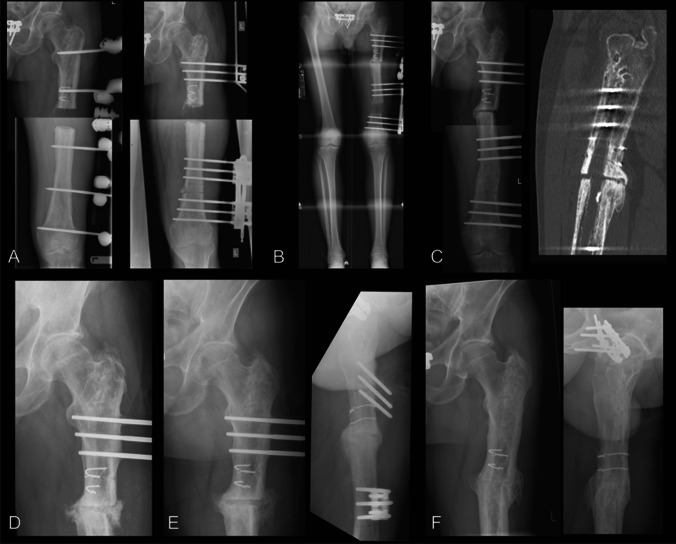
Docking site non-union in patient treated for infected non-union of femur managed by bone grafting. A Initial radiographs following removal of femoral nail and debridement (left). A monolateral external fixator is applied for bifocal bone transport with partial shortening (right). B Standing alignment films show transport and re-lengthening is complete. C Distraction segment has consolidated but docking site appears to only have tenuous union at best. Simple compression has been applied follow by compression and distraction (accordian technique). CT confirms docking site non-union. D Patient undergoes docking site procedure with freshening of the bone ends and application of autologous bone graft along with bone marrow aspirate concentrate and bioactive glass graft expander. E At 4 months post grafting, the docking site appears to have united, and the fixator is removed. F Radiographs at 12 months post fixator removal show union and remodelling of the docking site
